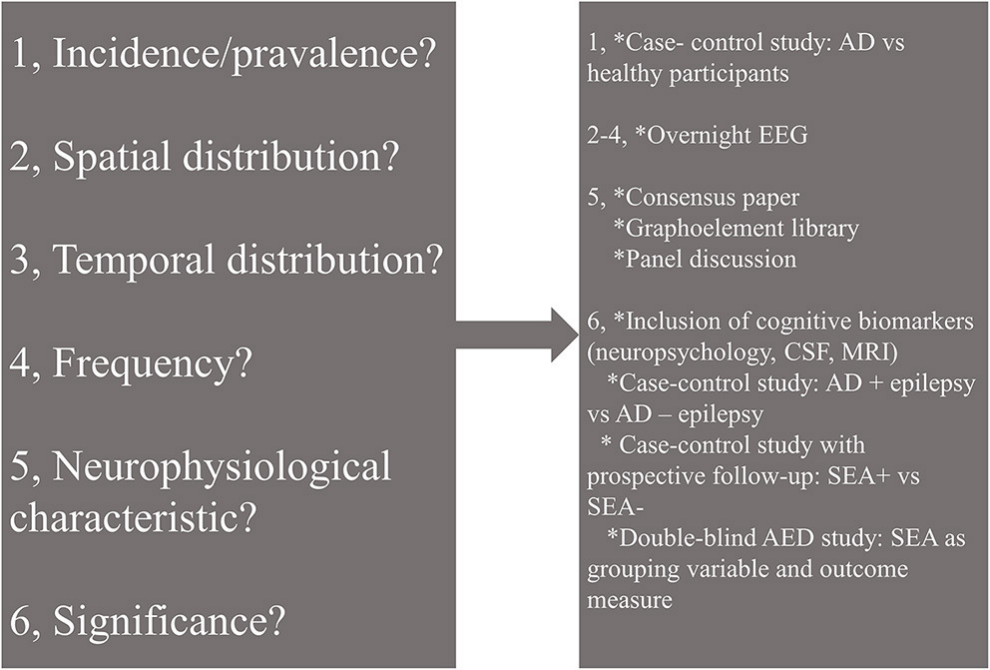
Open questions (left) and possible solutions (right) in the research of subclinical epileptiform activity (SEA) in Alzheimer's disease. AD, Alzheimer's disease; EEG, electroencephalography; CSF, cerebrospinal fluid; MRI, magnetic resonance imaging; AED, antiepileptic drug; SEA, subclinical epileptiform activity.
